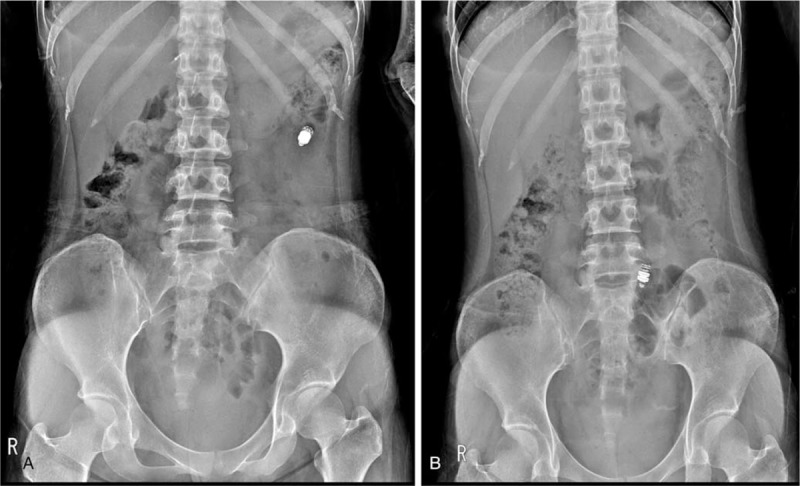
Serial abdominal radiographs after capsule endoscopy. (A) Radiograph 2 weeks after capsule endoscopy shows capsule retention in the left upper quadrant. (B) Radiograph 3 months after capsule endoscopy shows capsule retention in the lower abdomen.
